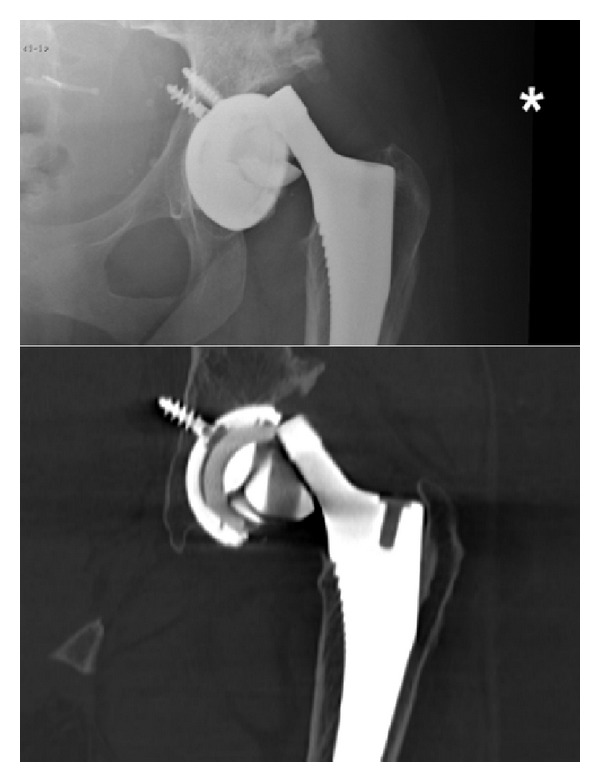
Plain radiograph and CT scan demonstrating fragmentation and dislocation of the ceramic head.
